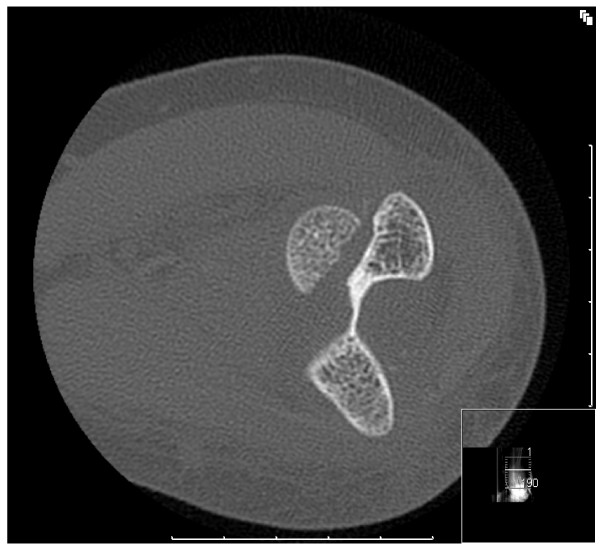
CT scan of capitellar fracture.
